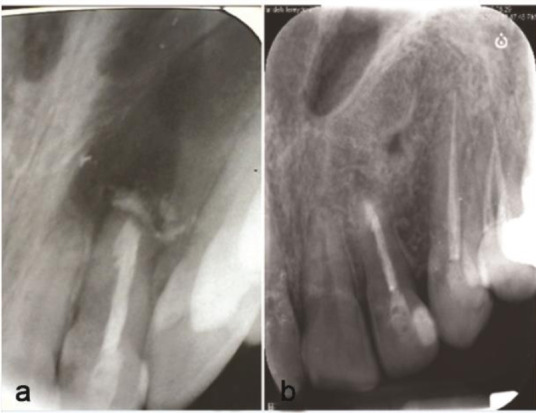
a: Surgical retreatment, b: Follow-up image after 6 years; the periapical radiolucency has disappeared
